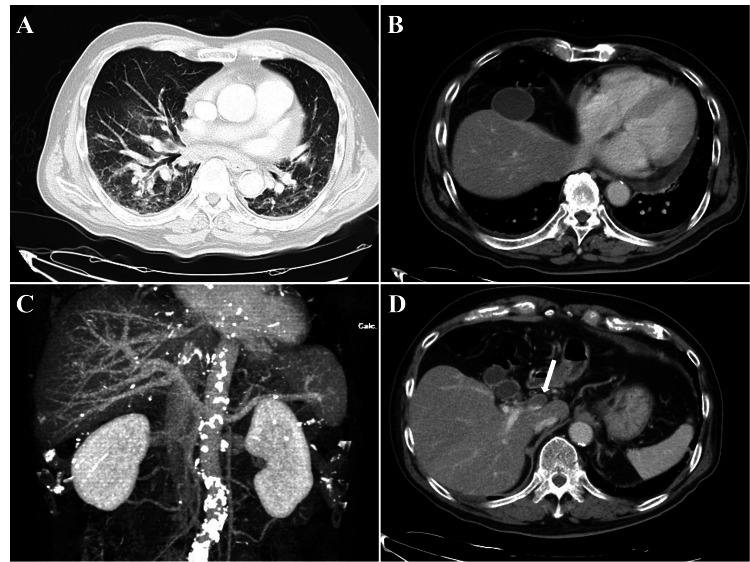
Contrast-enhanced CT on initial presentation (A) Chest CT reveals frosted shadows, and thickened interlobular septal walls in the lung bases bilaterally. (B, C) Abdominal CT reveals the disappearance of the left lobe of the liver and (D) defect of contrast effect in the left portal vein on admission (white arrow) CT: computed tomography
